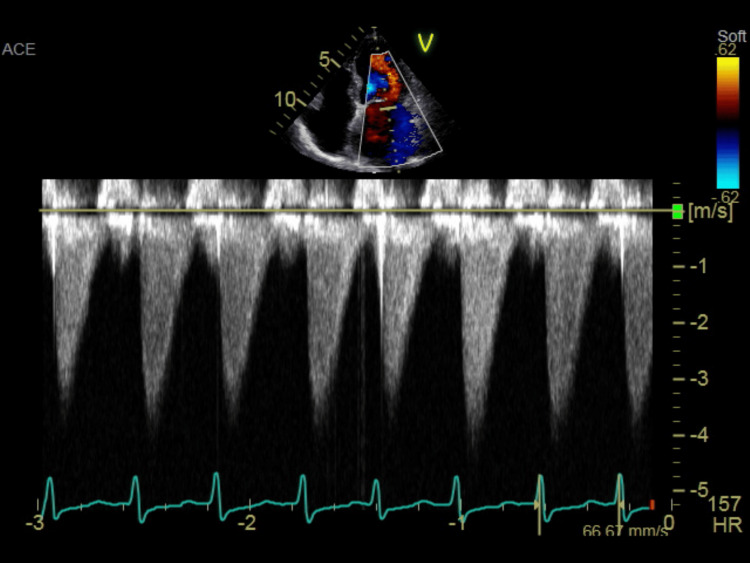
Continuous-wave Doppler during an acute episode. Continuous-wave Doppler of the MR jet demonstrates a characteristic dagger-shaped contour with an early-systolic sharp peak followed by rapid deceleration. MR, mitral regurgitation.
